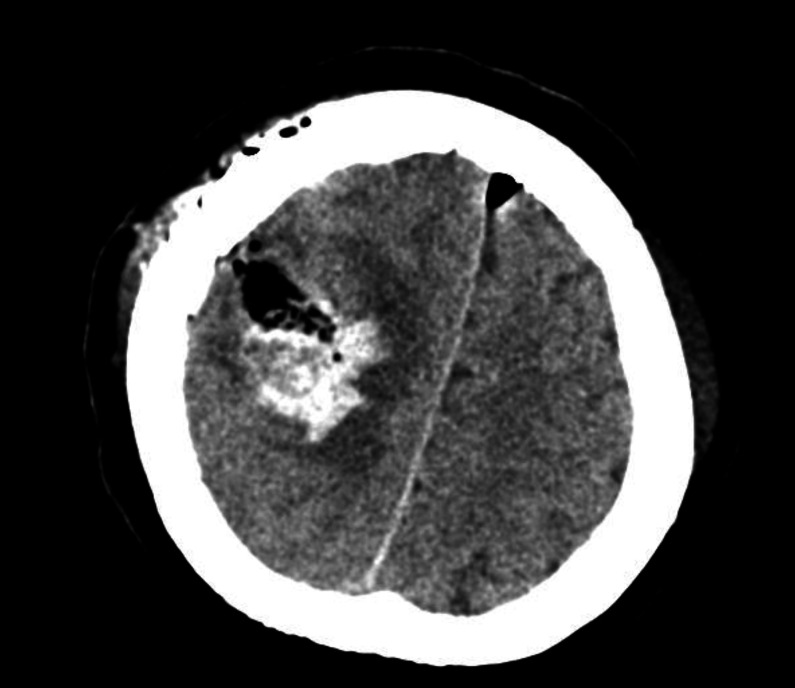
Brain CT scan. A heterogeneous intraparenchymal cerebral hemorrhage in the right frontoparietal lobe with surrounding edema
